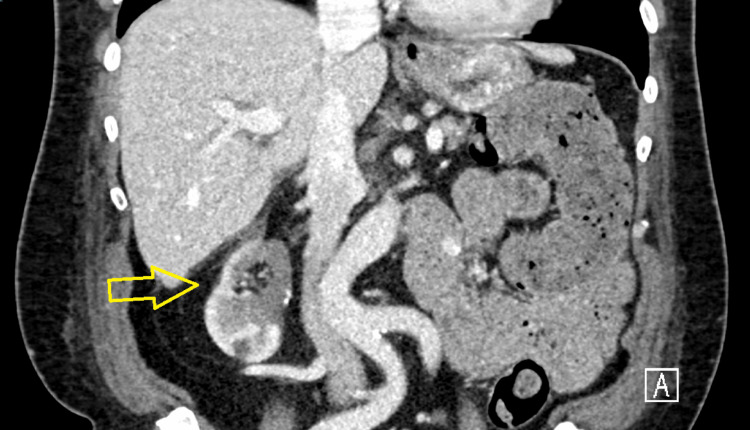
CT of the abdomen/pelvis showing the right partial nephrectomy site with postoperative changes and no abscess.
